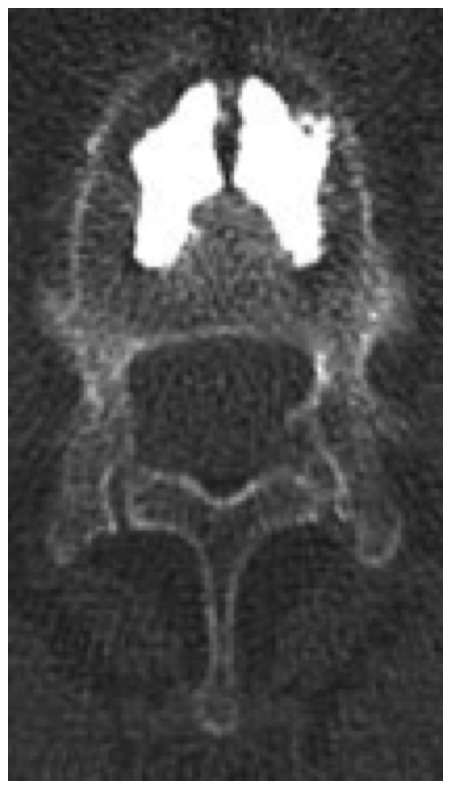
Case presentation with Osseofix® system. Radiological case report. Axial slice CT of L1 after stabilization with Osseofix® system.
